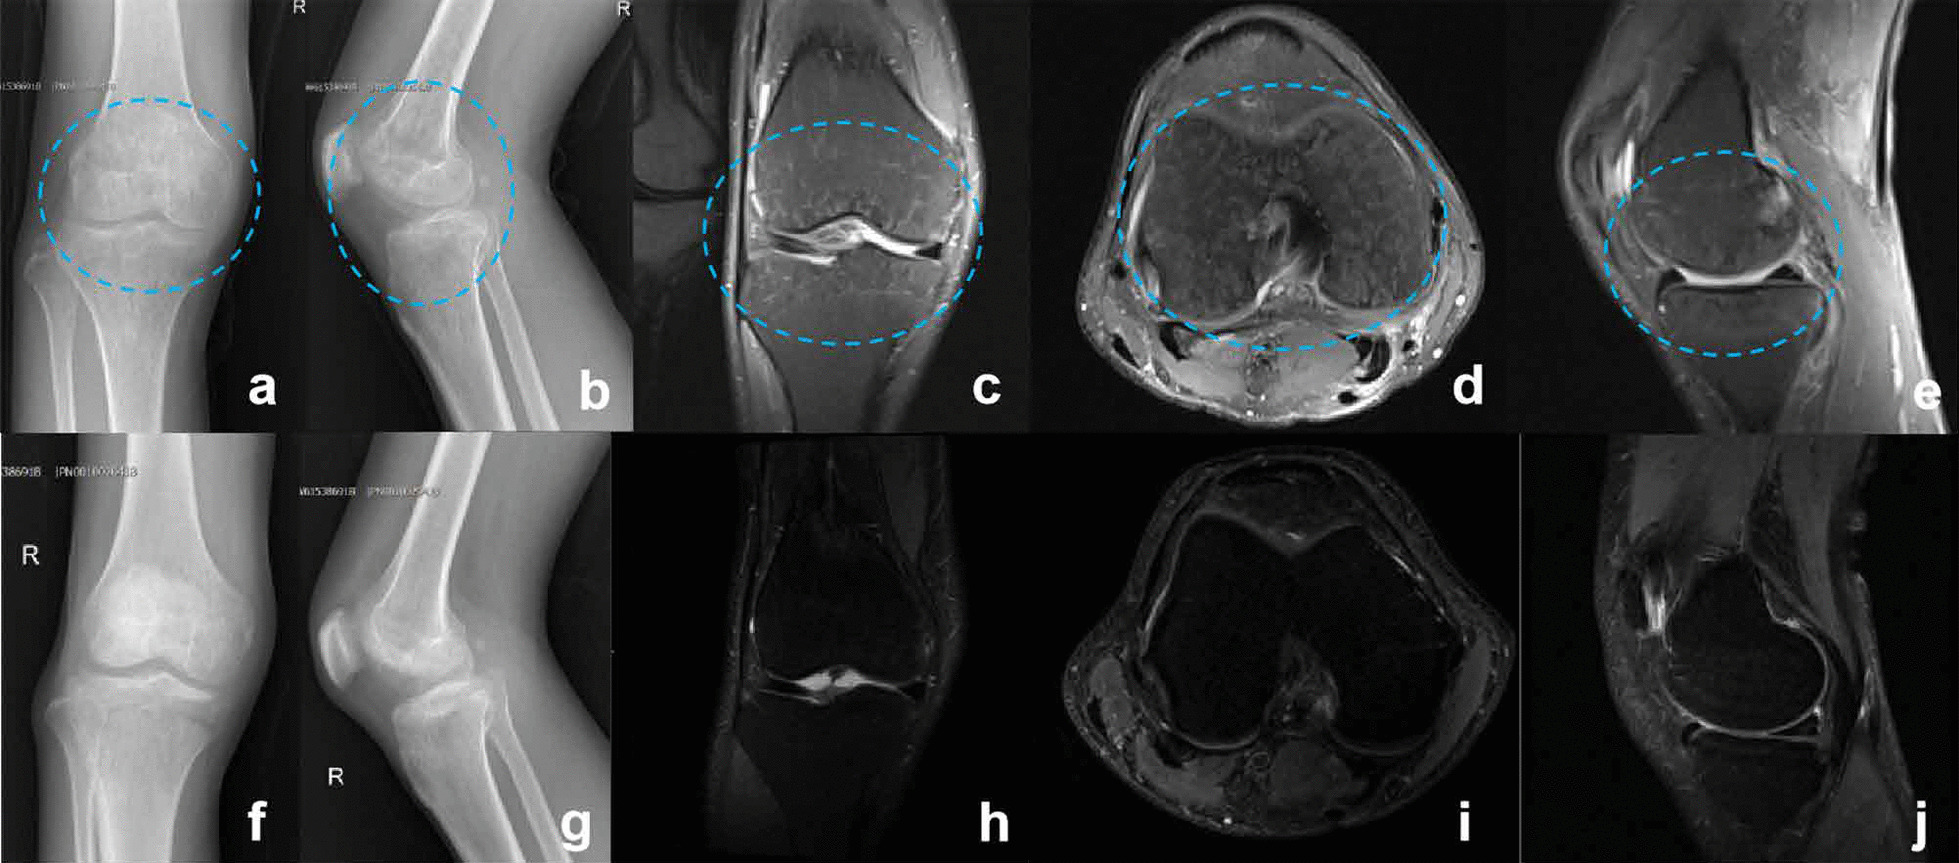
Patient 1: X-ray images showed multiple cystic low density areas (blue circle) of distal right femur and proximal tibia (a, b). PDWI sequence of MRI showed effusion and synovial thickening of the right knee joint, and subchondral bone marrow edema (blue circle) of the distal femur and proximal tibia (c–e). Post-treatment X-ray images showed the low density area was significantly reduced (f, g). Post-treatment PDWI sequence of magnetic resonance images indicates the high signal area of bone marrow edema had disappeared (h–j)
